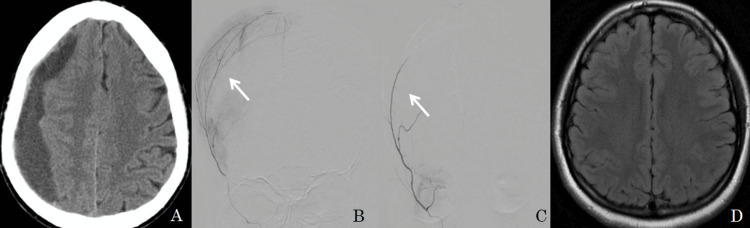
A 65-year-old male presented with right-side chronic SDH (image A). On selective catheterization of the right MMA shows tortuous frontal and parietal branches and abnormal blush (image B). Embolization with embospheres was performed and a post-embolization angiogram (image C) shows an absence of blush and a follow-up MRI after 6 months (image D) shows complete resolution.
